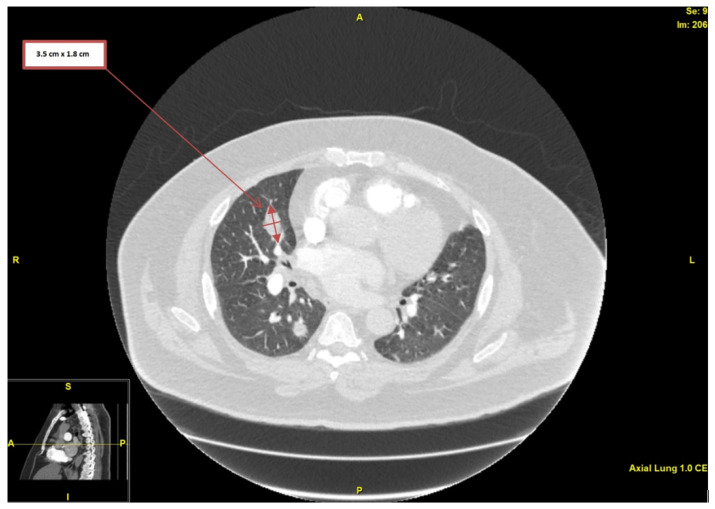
Computed tomography angiography of the chest, showing nodularity with associated cavitation (largest measuring 3.5 × 1.8 cm in diameter).
